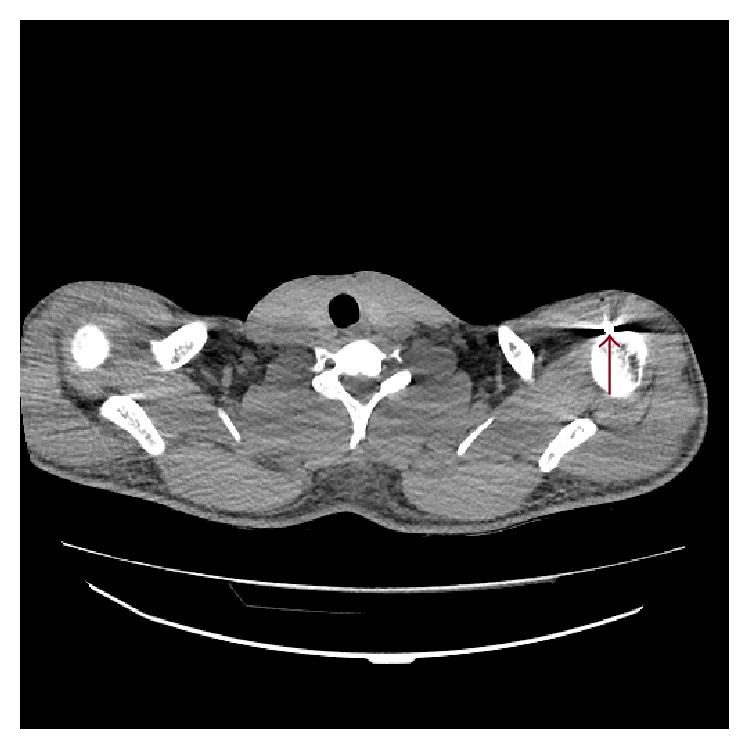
Transverse cut view of chest CT shows a bullet in the left shoulder at proximal humerus.
